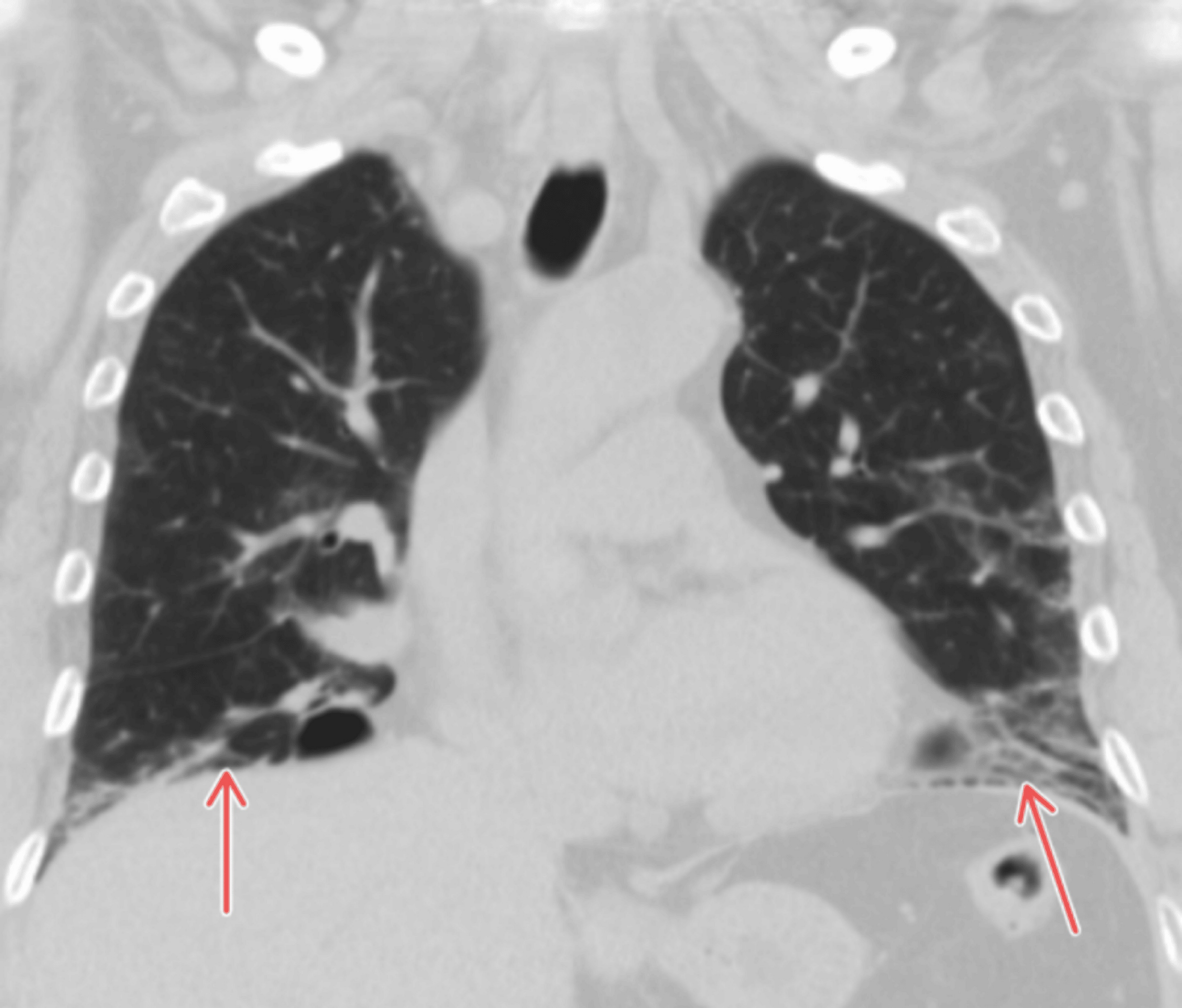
Coronal HRCT in 2025 demonstrating bibasilar fibrotic scarring (red arrows) with reticulation and mild traction bronchiectasis, most consistent with a fibrotic nonspecific interstitial pneumonia pattern in connective tissue disease-associated ILD. HRCT: high-resolution computed tomography; ILD: interstitial lung disease
